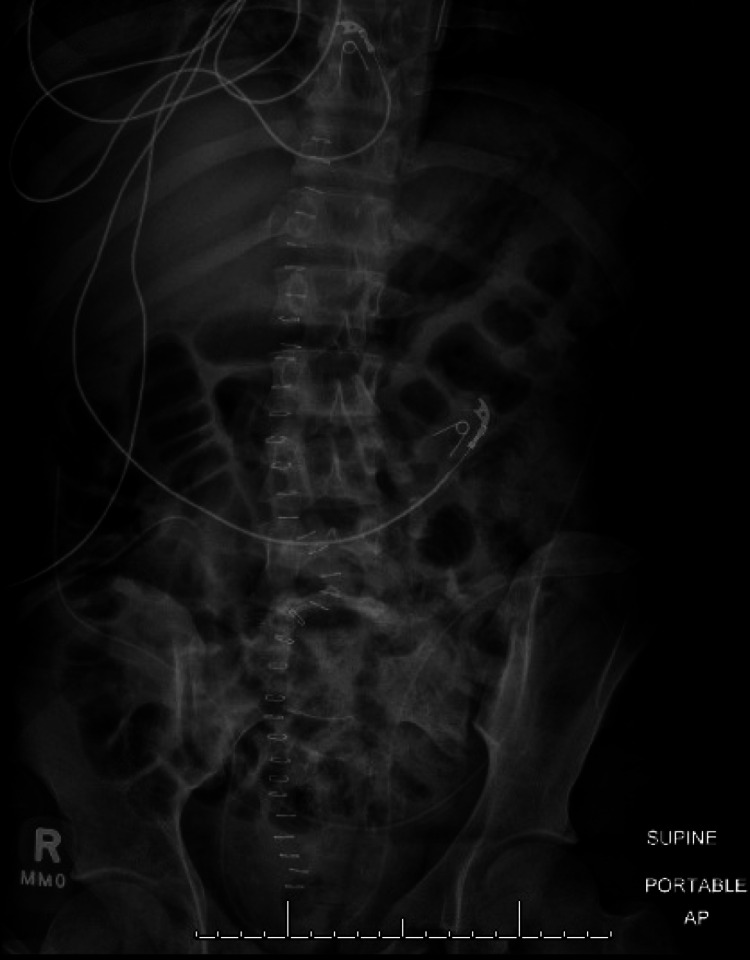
Pre-operative Sacral Fracture Anteroposterior X-ray from outside hospital demonstrating pre-sacral screw placement fracture
